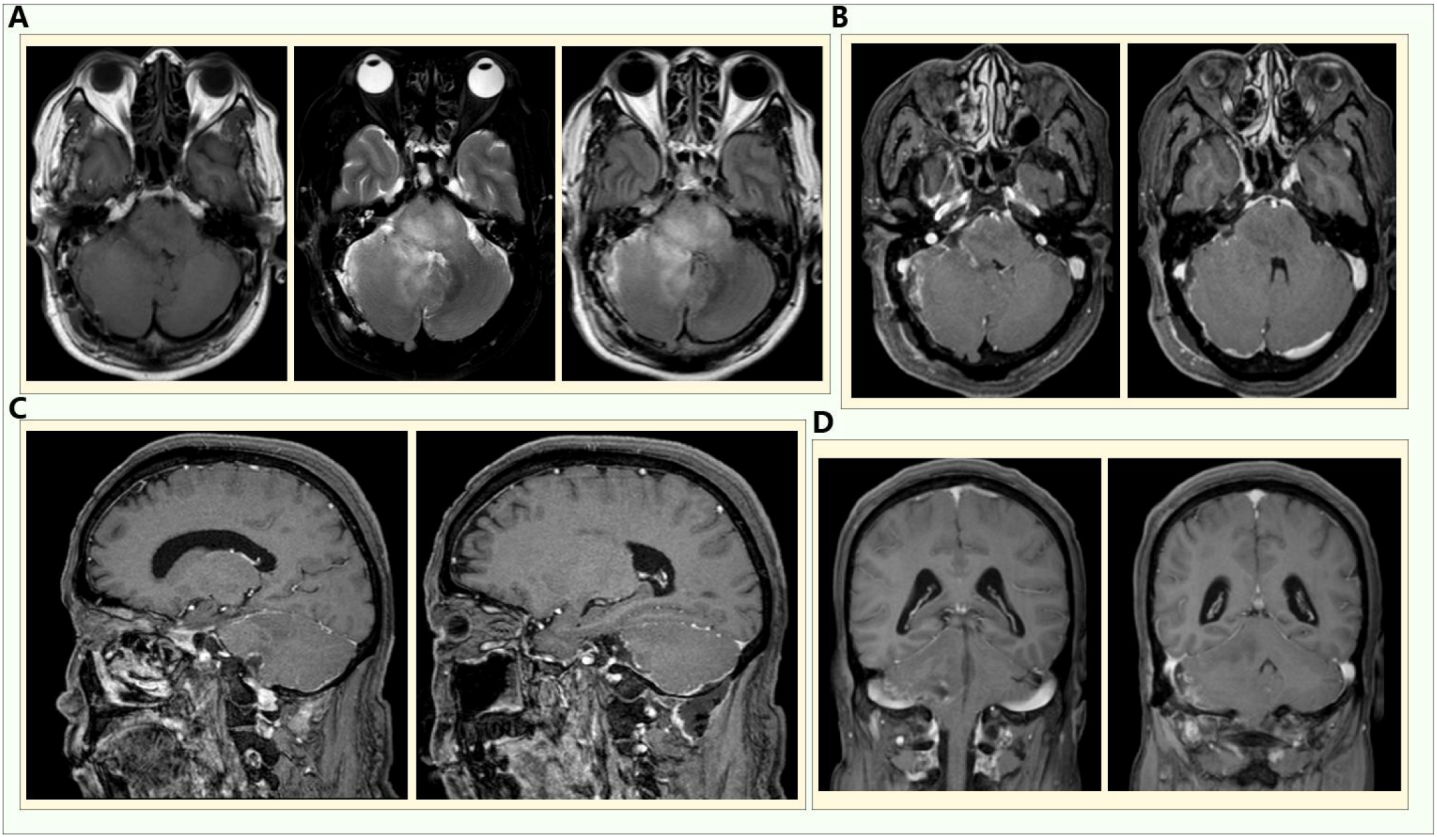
Head enhanced MRI of the patient after the operation. (A) The T1, T2 and FLAIR sequences respectively; (B-D) the axial position, sagittal position and coronal position of the enhanced scan.
